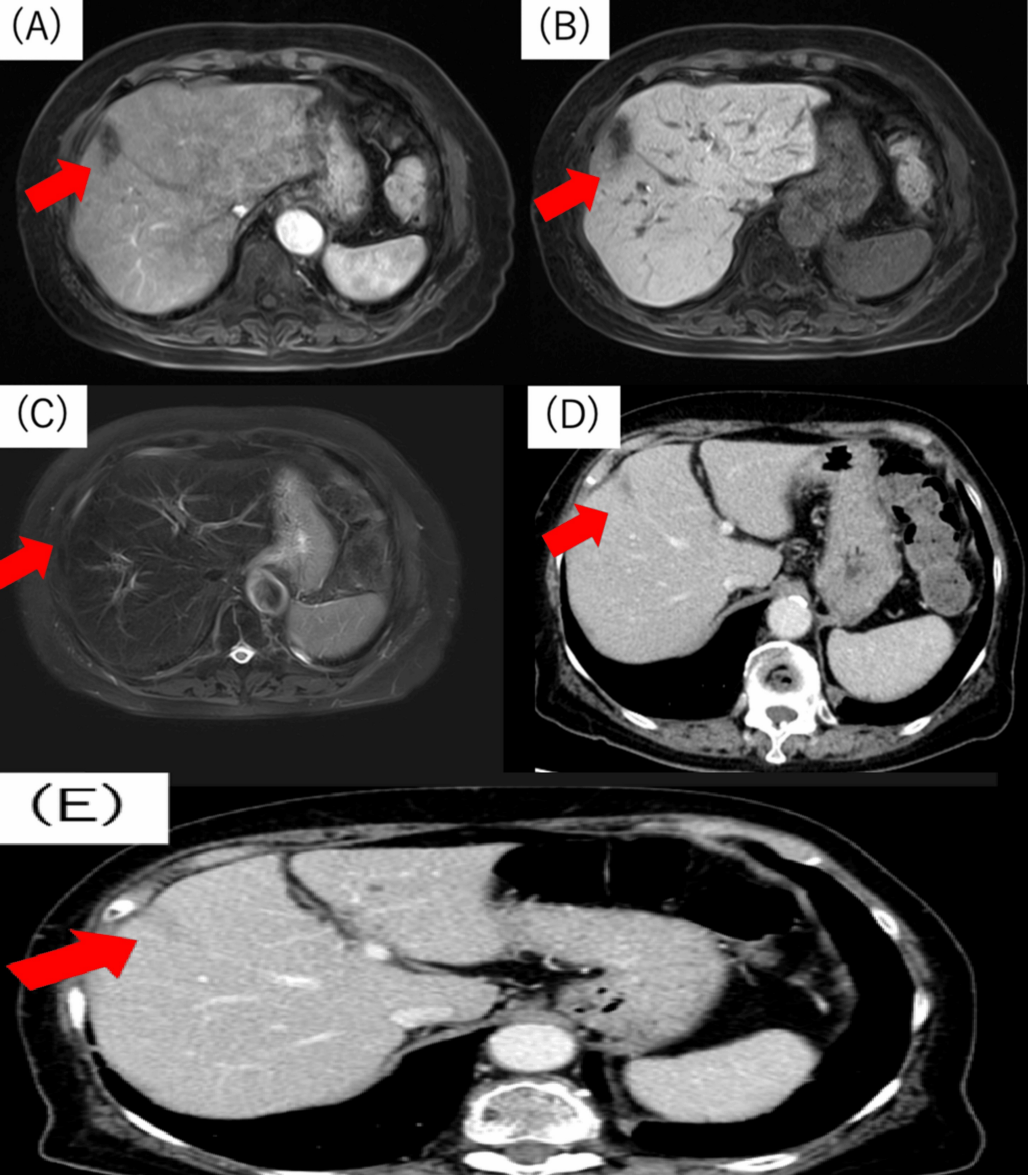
Findings from a follow-up MRI scan performed during hospitalization, CT image taken at discharge, and the outpatient follow-up CT. (A) In the arterial phase of the MRI, no evident contrast enhancement is observed (arrow). (B) In the hepatocyte phase of the MRI, a 22-mm hypointense area was observed, accompanied by a faint hypointense signal in the surrounding region (arrow). (C) In the T2-weighted imaging of the MRI, an isointense signal was observed (arrow). (D) Contrast-enhanced CT confirmed the disappearance of the abscess (arrow) (on day 51). (E) The abscess had resolved by the time of the outpatient consultation after discharge (arrow) MRI: magnetic resonance imaging; CT: computed tomography
